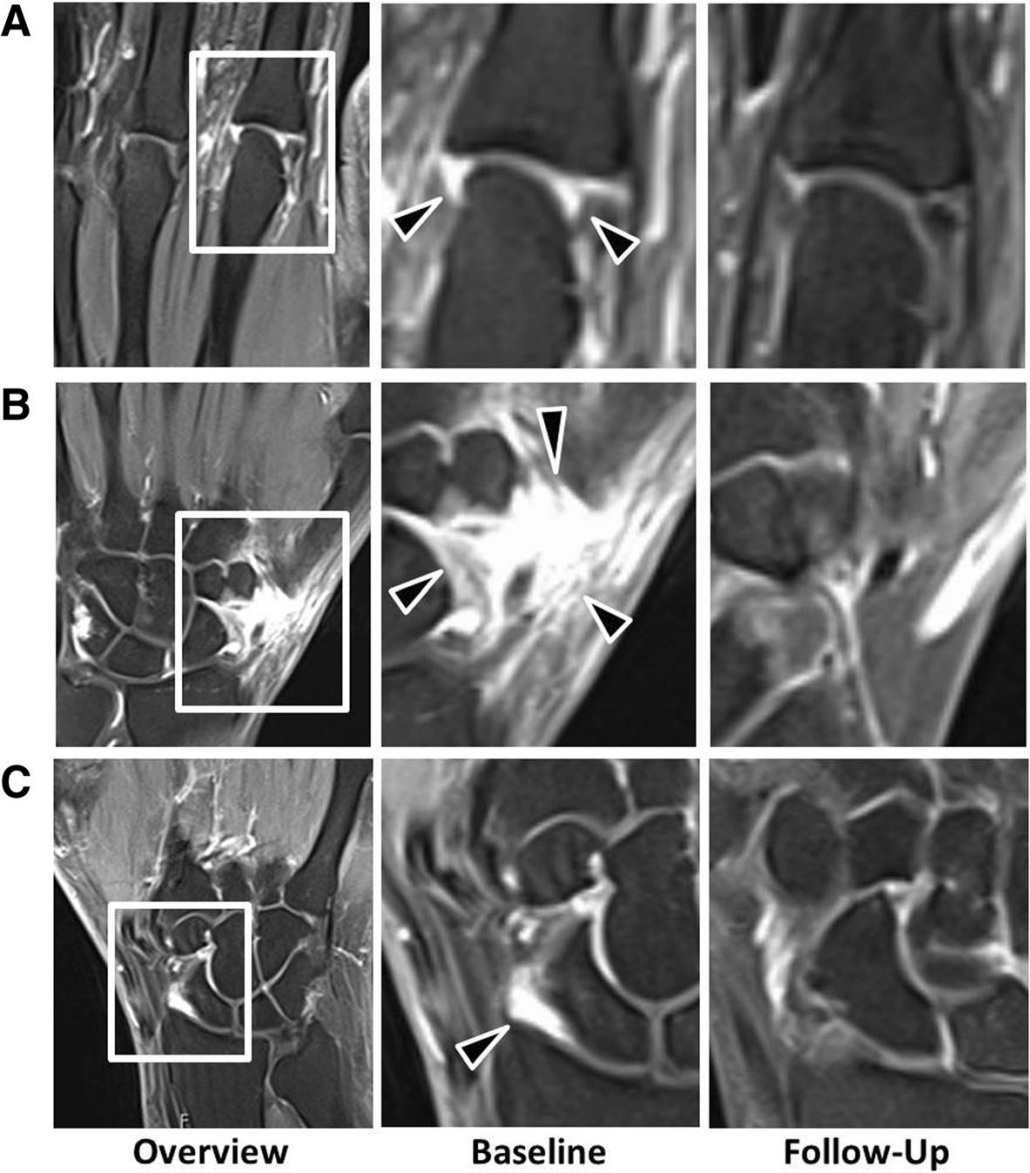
a–c Effects of secukinumab on signs of MRI inflammation in psoriasis patients at risk to develop PsA. T2-weighted coronal fat saturated (TIRM) sequences of the hands of psoriasis patients at risk to develop PsA. Three examples, one from metacarpophalangeal joints and two from the wrist joint, are shown. Left column: overview at baseline; middle column: close-up of the inflammatory lesion at baseline; right column: follow-up of the same region after 24 weeks of secukinumab treatment. Black arrows mark the lesion; white frames mark the area depicted in the close-up
